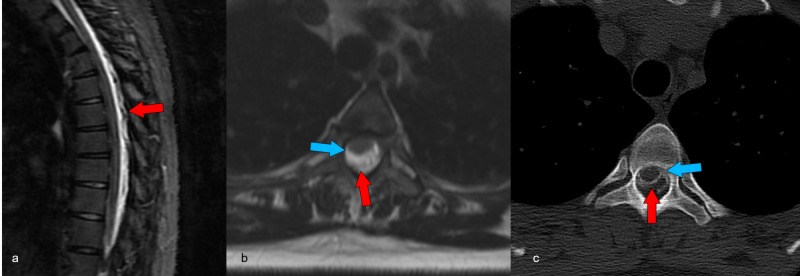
Spinal epidural hemorrhage The sagittal T2-weighted MRI image reveals (a) posterior epidural fluid collection with loculations compatible with a mixture of CSF leak and epidural hematoma (red arrows). A corresponding axial T2-weighted image (b) at T3–T4 also demonstrates this finding. There is effacement and displacement of the thecal sac and cord anteriorly (blue arrow). Axial CT myelogram image at T3–4 (c) shows epidural contrast with superimposed epidural hemorrhage causing effacement and displacement of the thecal sac and cord anteriorly as seen on MRI. MRI: magnetic resonance imaging, CSF: cerebrospinal fluid, CT: computed tomography
